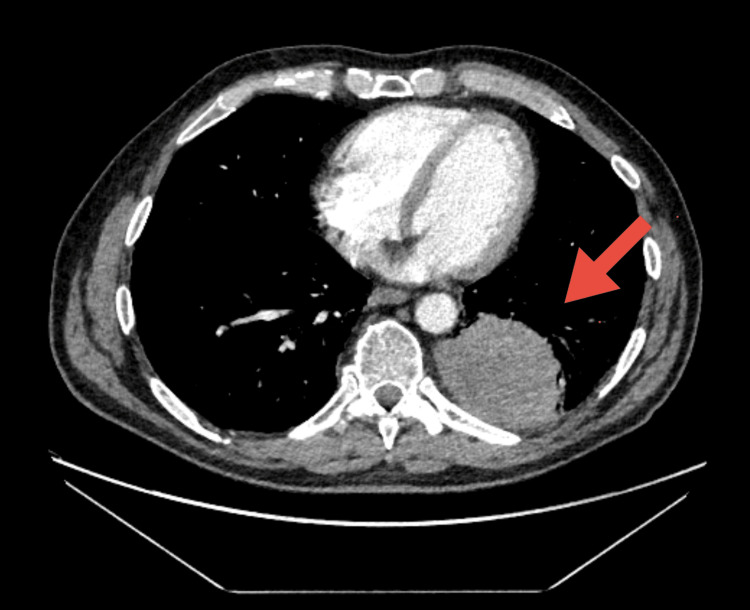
CT thorax showing a 2.8 cm left lower lobe mass (arrow) abutting the posterior pleura
